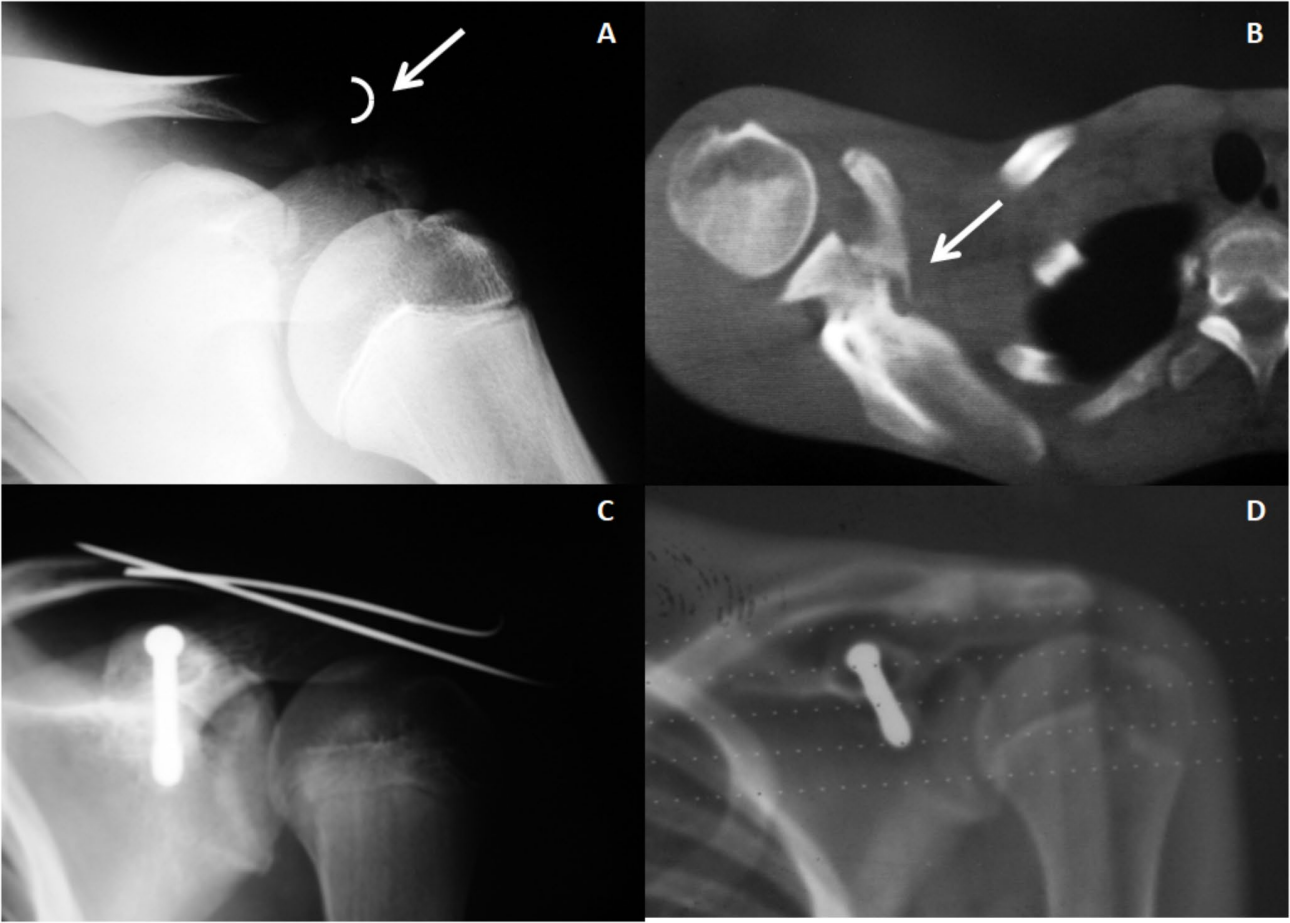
Case 2 Table 1. A 16 years-old boy: 1A: Antero-posterior radiograph showing an AC separation (white arrow), there is no clear vision of the CP; 1B: A CT scan shows a type I base associated CP fracture; (white arrow indicating the limits of the distal end of the clavicle drawn in white); 1C: Surgical treatment was performed with a screw fixation of the CP fracture and AC temporary fixation with Kirschnner wires; 1D: Radiological appearance at three months follow-up
